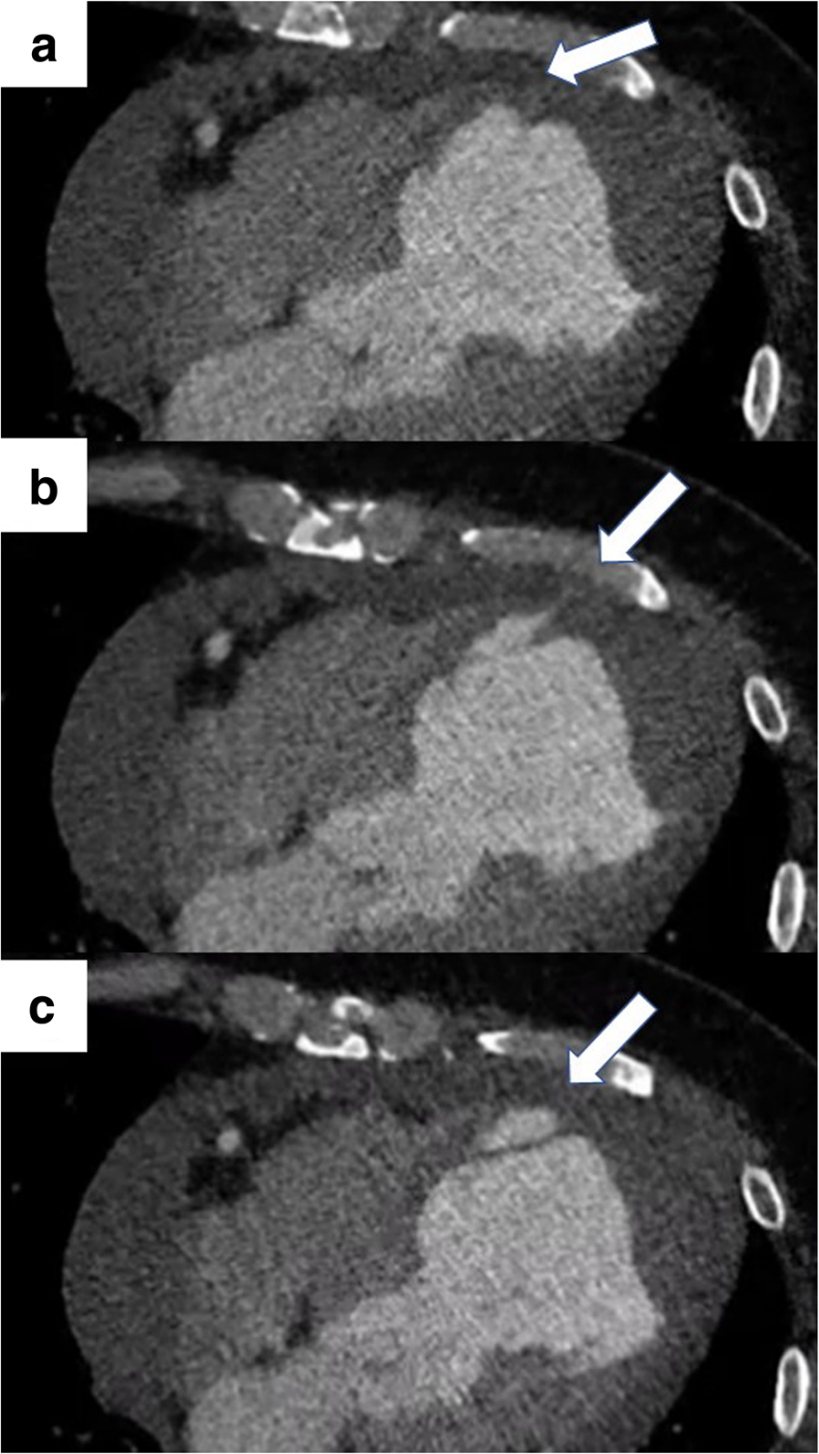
Preoperative computed tomography. (a–c) Extensive aneurysm formation on the anterior LV wall, with pericardial effusion accumulation. (b) The aneurysm showing leakage of contrast from the inner cavity to the LV myocardium. (c) Intra-LV wall aneurysm with the dissected myocardium
